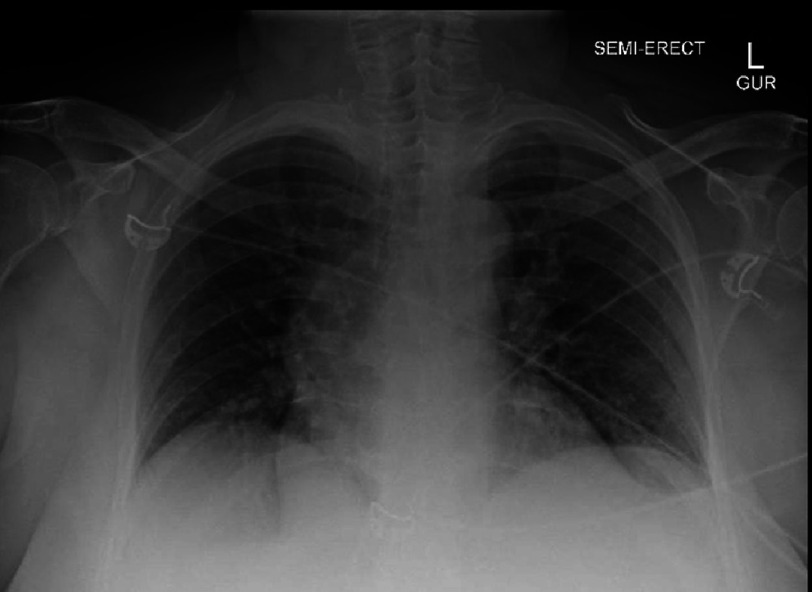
The chest X-ray showing a widened mediastinum.
